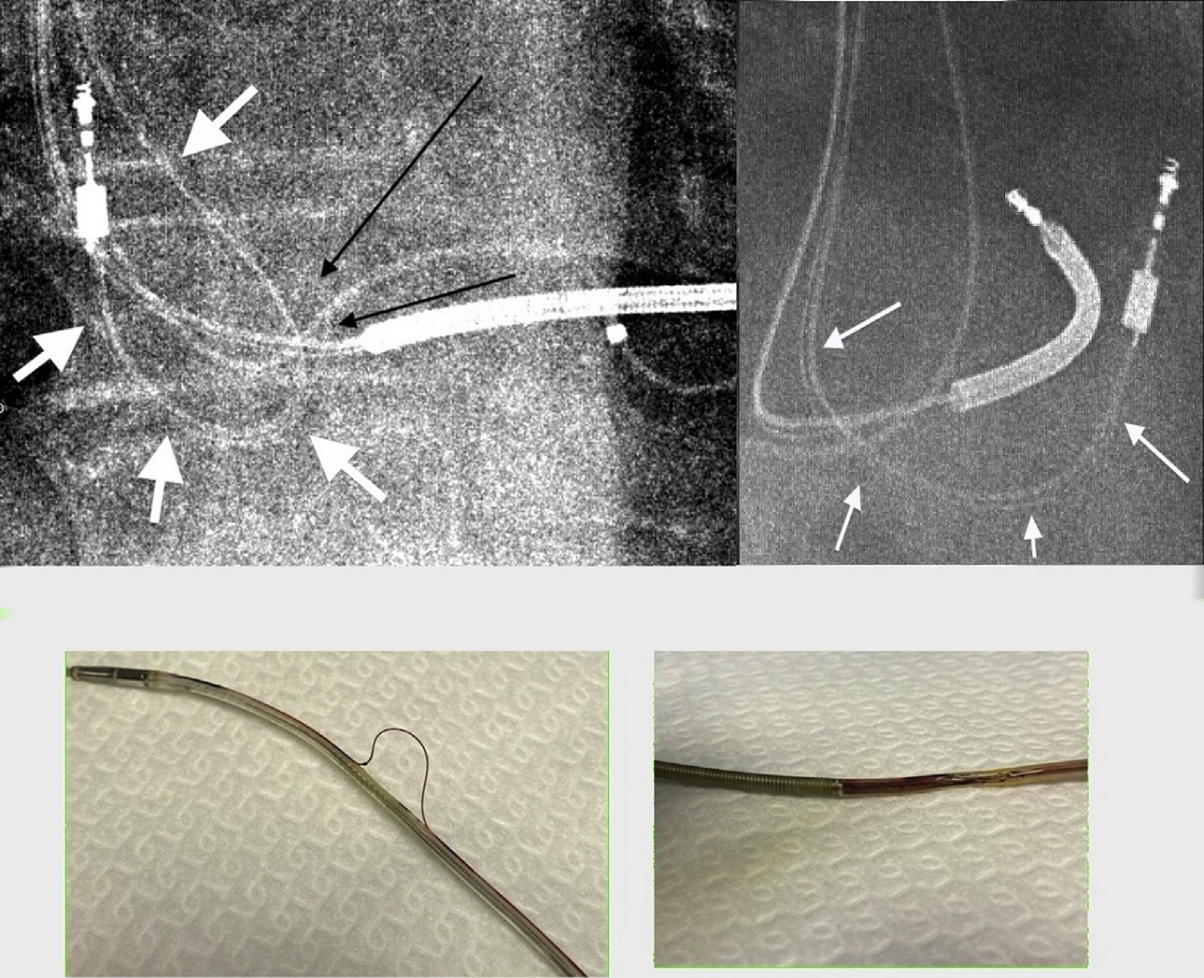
Upper: Anterior-posterior and lateral views of chest radiographs. Leads appear to be normally placed, although the atrial lead was making a loop, evoking excessive slack, descending very low in the right atrium probably neighboring the tricuspid valve (*white arrows*). Cautious inspection reveals conductor externalization (*black arrow*). Lower: Insulation breaches of atrial (left) and right ventricular (right) leads, with the atrial lead additionally showing an externalized conductor.
